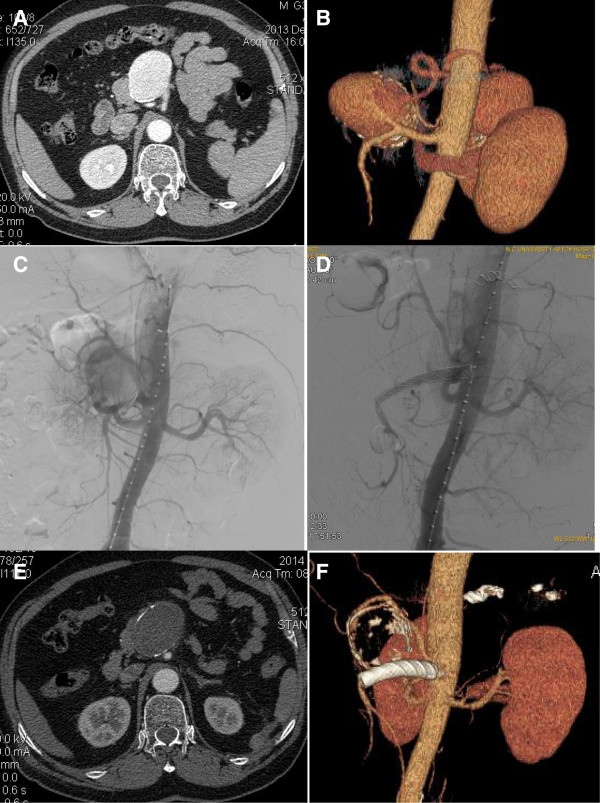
The image finding of case four during preoperation, intraoperation and follow-up. A, B and C, Preoperative CTA and DSA demonstrated a 49 mm × 59 mm SAA near the origin of the SMA and the proximal neck of the SAA was 5 mm. D, The completion aortogram revealed no evidence of endoleak; disappearance of the aberrant splenic artery aneurysms lumen and patency of the SMA. E and F, The CTA in 6 months postoperatively demonstrated that the SAA sac was thrombosed with marked shrinkage; the SMA is patent with no infarction or abscess formation in the spleen.
